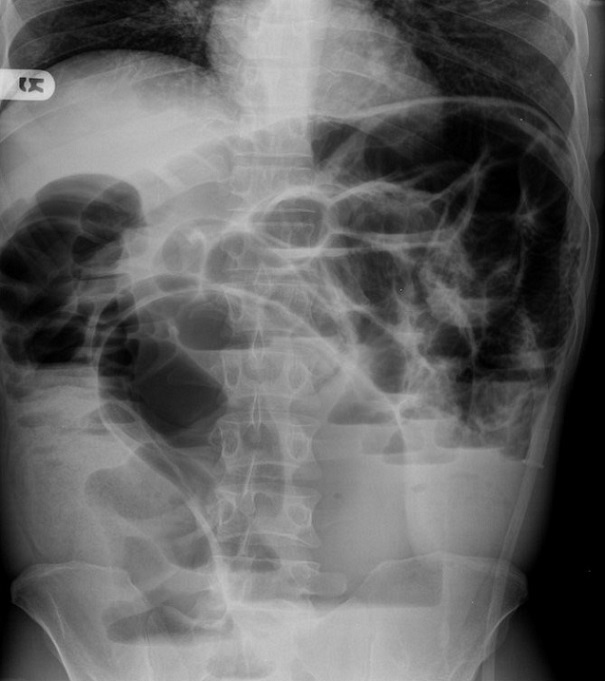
Plain upright abdominal X-ray showing dilated sigmoid colon and dilated small bowel with multiple air-fluid levels
